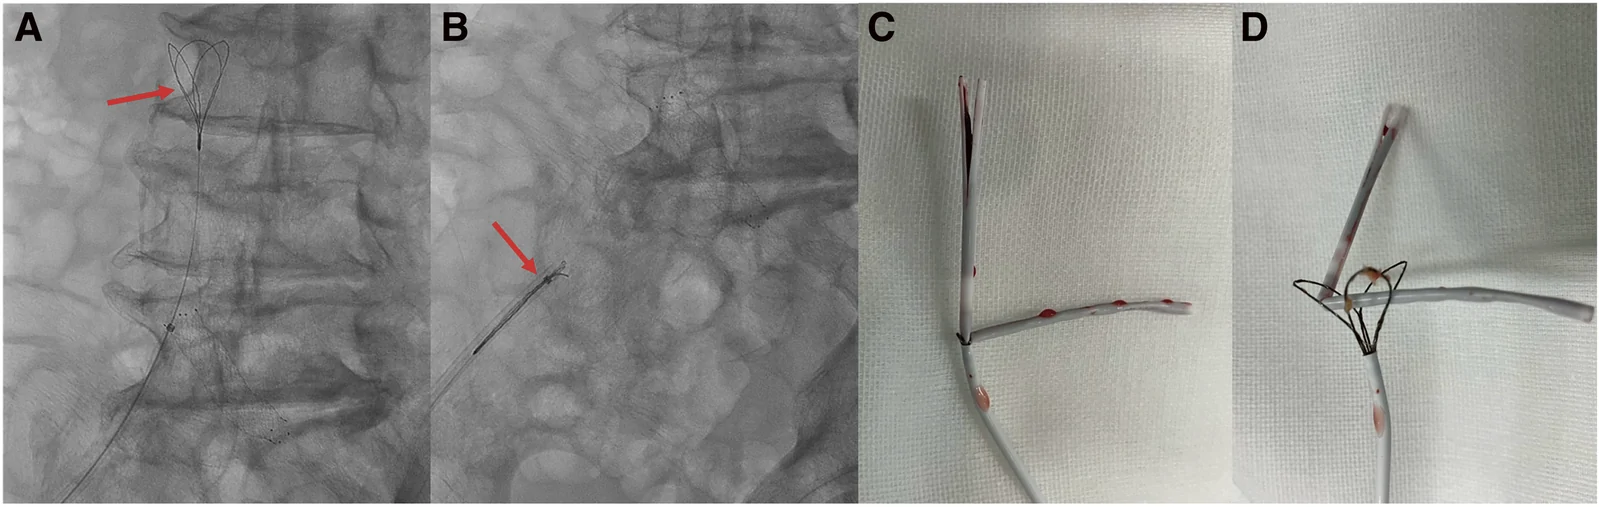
Abdominal radiographs and digital photos obtained during retrieval of the catheter straightener. (A) Radiograph showing the deployed EN Snare (arrow) at the level of the 3rd lumbar vertebra. (B) Pulling back of the EN Snare into the right femoral sheath (arrow). (C) Digital photo showing the retrieved catheter straightener and EN Snare. (D) Retrieved catheter straightener and an artificially partially deployed EN Snare.
